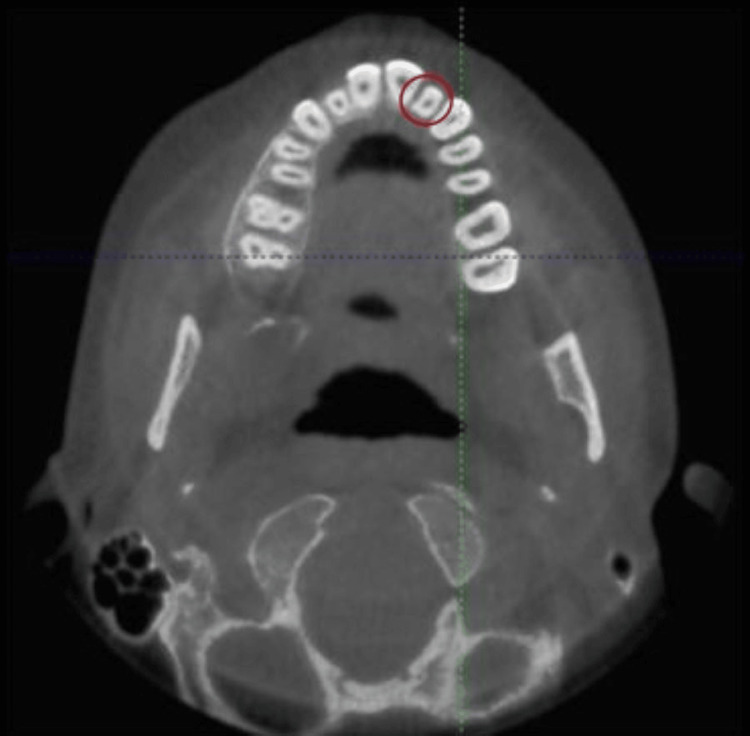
Axial CBCT image Axial CBCT section showing type I palatogingival groove with #22. CBCT: Cone beam computed tomography.
